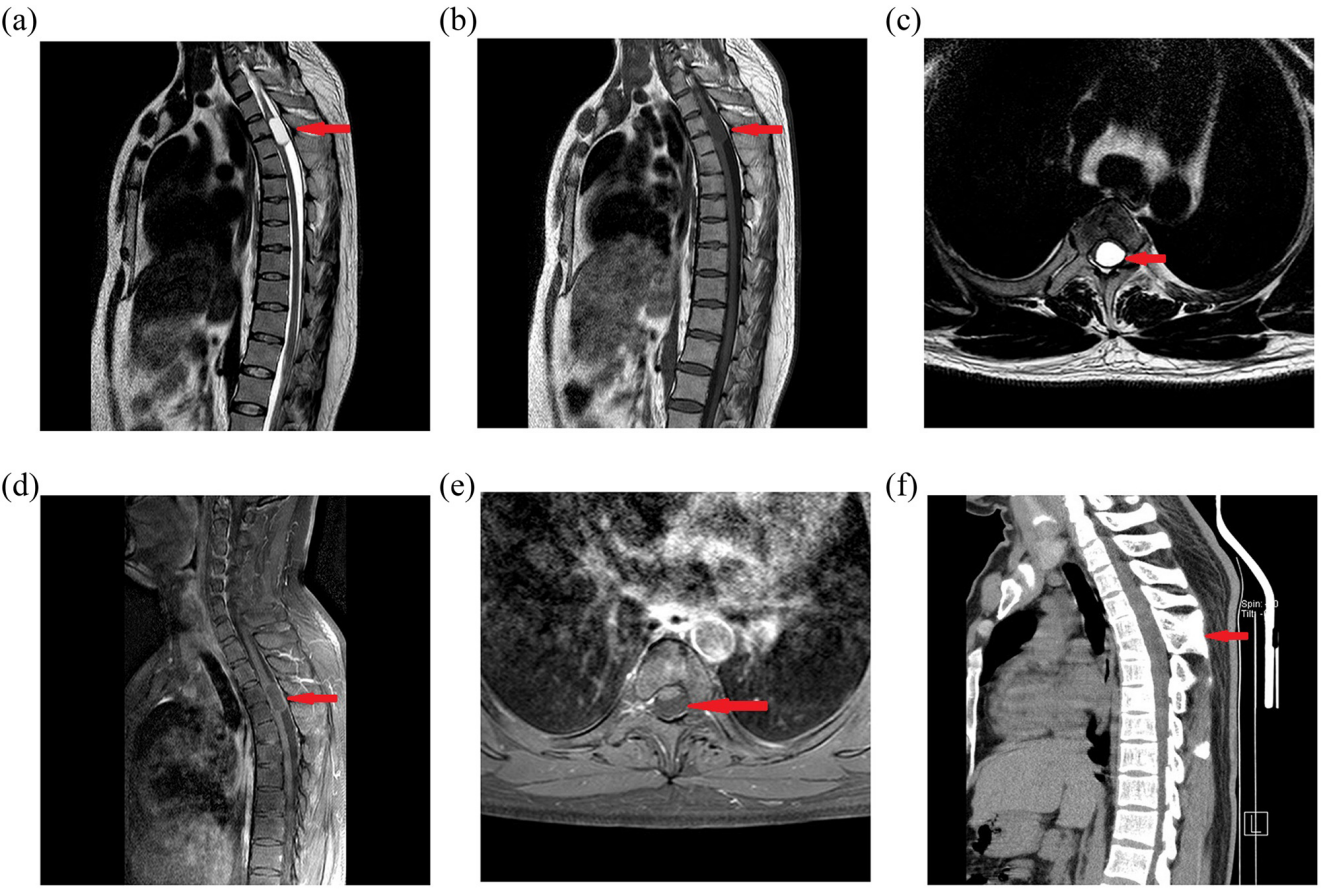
(a) T2 image of a sagittal plain MRI scan. On the third to the fourth thoracic vertebral plane, a cystic mass with significant signal intensity could be identified. It is evident that the spinal cord is compressed. (b) T1 image of a sagittal plain MRI scan. On the third to the fourth thoracic vertebral plane, a cystic mass that is T1 isointense or slightly hypointense is visible. (c) A horizontal T2 image from a plain MRI scan. The left dorsal side of the spinal cord has a cystic mass, and the spinal cord is compressed. (d) Enhanced sagittal plain MRI image. An enhanced cystic mass can be seen. (e) Enhanced sagittal plain MRI image. There is no visible cystic space occupying the enhancement. (f) Sagittal plain CT image showing the third to the fourth thoracic vertebrae and spinous process fusion.
